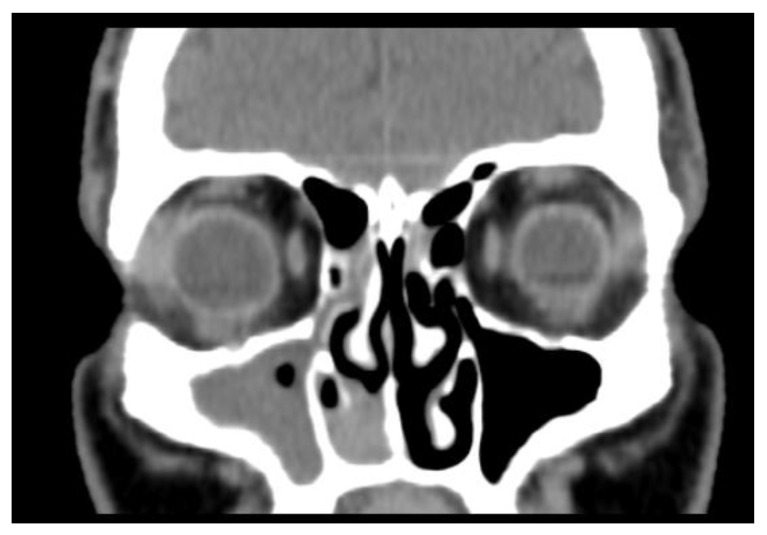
Preoperative CT scan showing a nonspecific opacification of the right nasal fossa (inferior meatus) with partial erosion of the inferior turbinate. There was no evidence of invasion into the skull base, cribriform plate, or olfactory cleft. The CT scan demonstrated density and mucosal thickening of the maxillary sinus.
